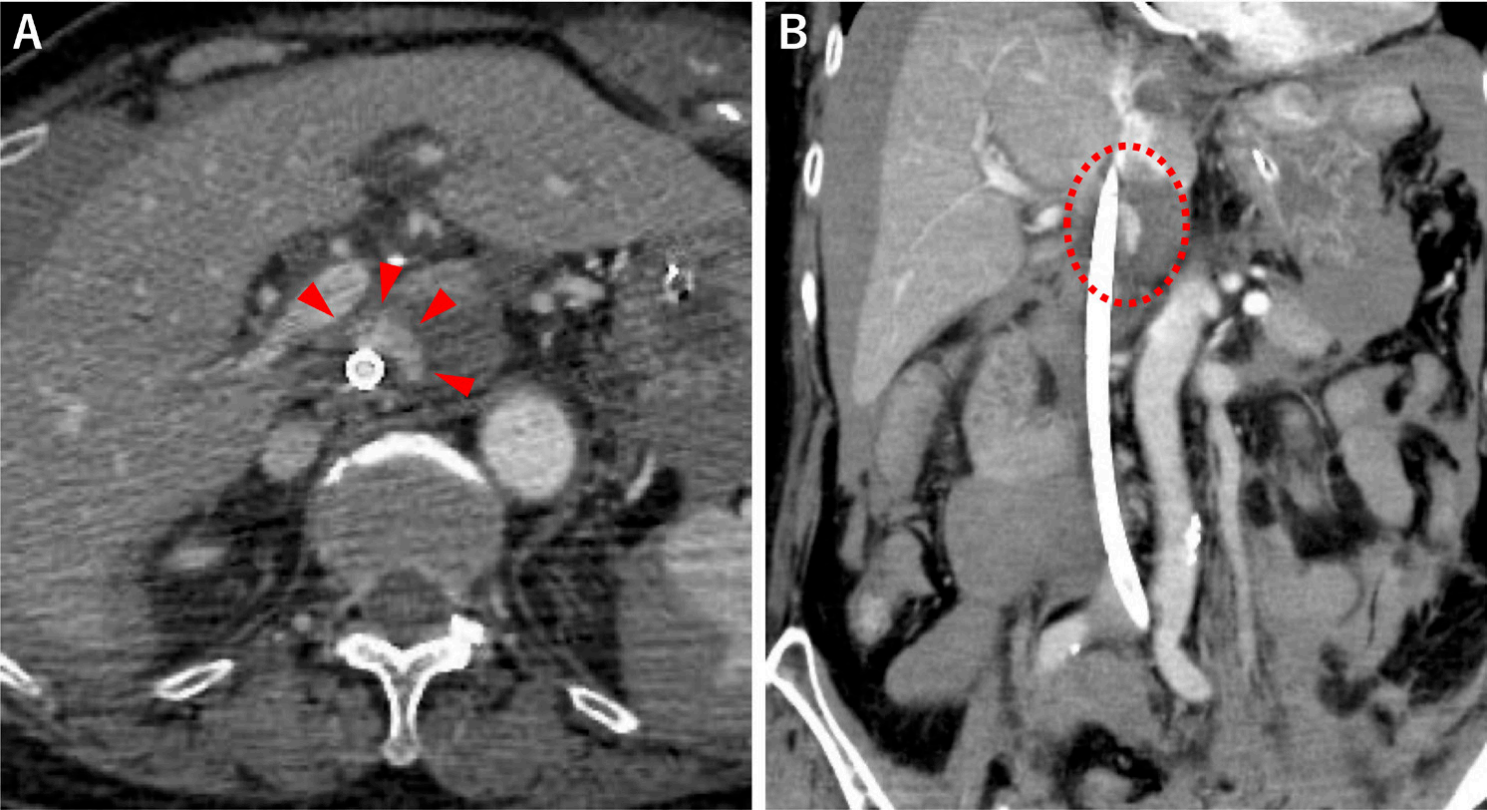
Contrast-enhanced CT after initiation of ECMO. (A) Axial view, (B) Coronal view. A 20-Fr drainage cannula for ECMO was inserted from the femoral vein to the right atrium. Contrast-enhanced CT showed extravasation of contrast medium around the IVC ((A) arrowhead, (B) dotted circle), suggesting retrohepatic IVC injury. The drainage cannula was located across the injured area. CT, computed tomography; ECMO, extracorporeal membrane oxygenation; IVC, inferior vena cava.
